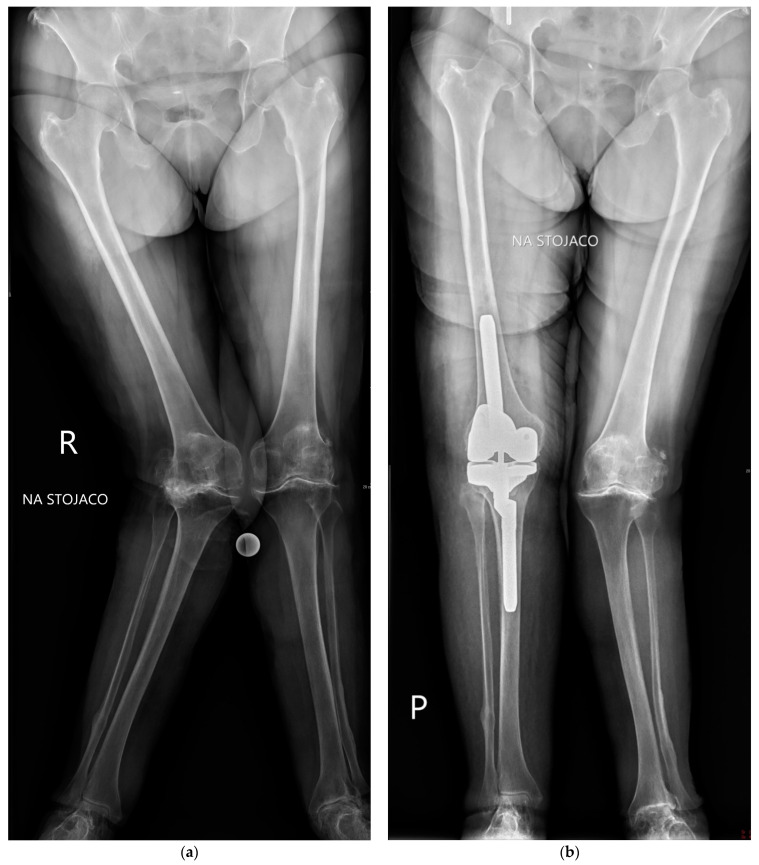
(a) Patient with grade III fixed valgus deformity’s long-standing X-ray. (b) Postoperative X-ray with mechanical axis correction.
